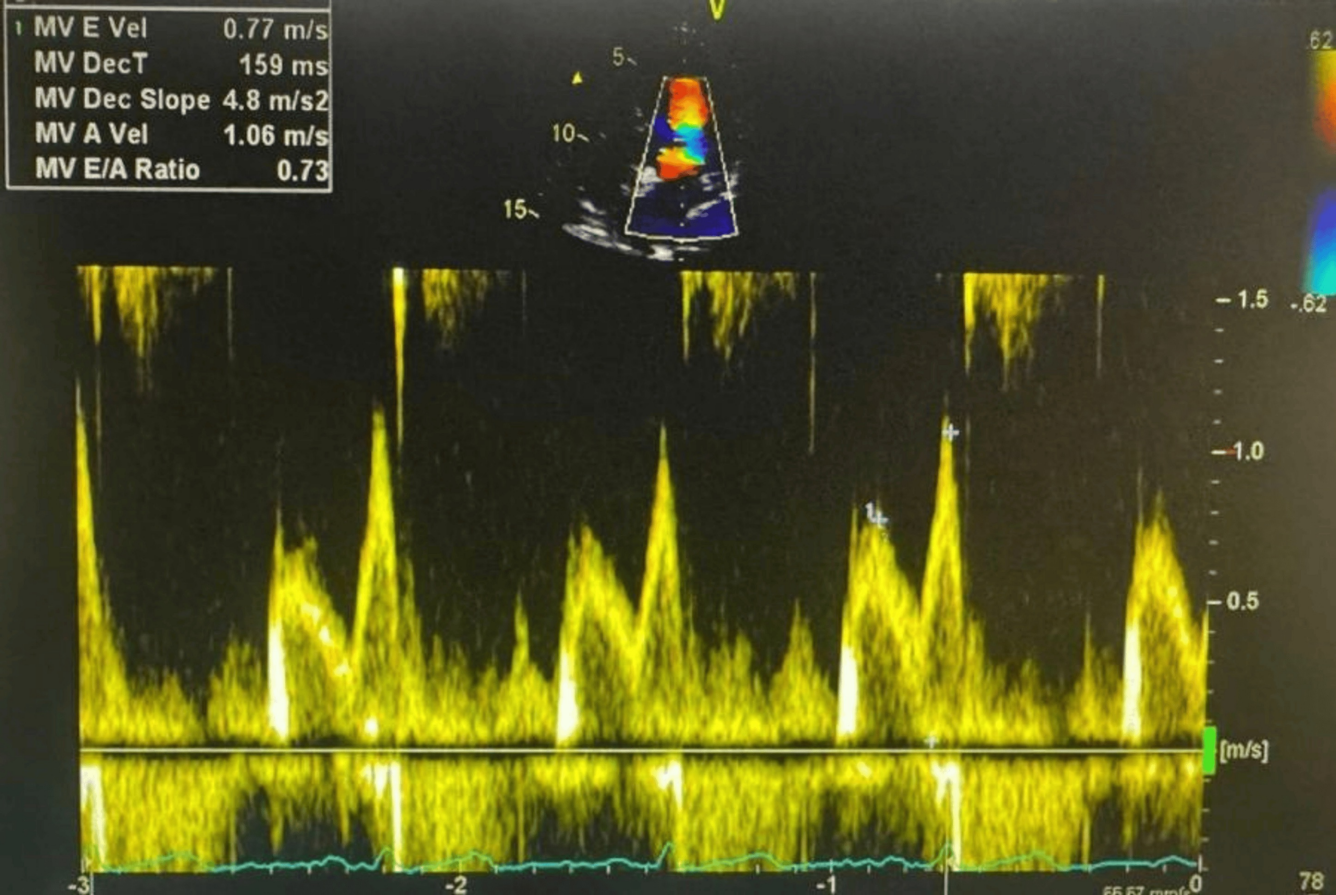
Pulse-wave Doppler echocardiography of mitral inflow showing an E/A ratio of 0.73 (E=0.77 m/s, A=1.06 m/s), consistent with grade I diastolic dysfunction (impaired relaxation pattern). MV E Vel: mitral valve E-wave velocity; MV DecT: mitral valve deceleration time; MV A Vel: mitral valve A-wave velocity; MV E/A ratio: mitral valve E/A ratio
